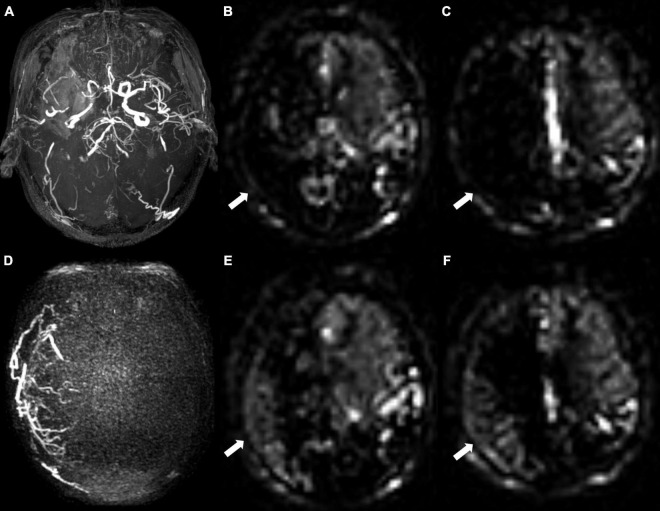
A 68-year-old male patient with unilateral moyamoya angiopathy. (A) Presurgical MR angiography image. Right middle cerebral artery was occluded. (B,C) Two-slice ASL images of ASPECT regions, and cerebral perfusion was significantly reduced. Preoperative collateral score was almost 0 in (B) and 1 in (C). (D) Postoperative super-selective 4D MRA images of external carotid artery. Postsurgical collaterals were defined as good (2 scores according to Matsushima standard). (E,F) ASL images at same level as (B,C). Postoperative collateral scores increased (white arrows), which were 6 in (E) and 6 in (F).
